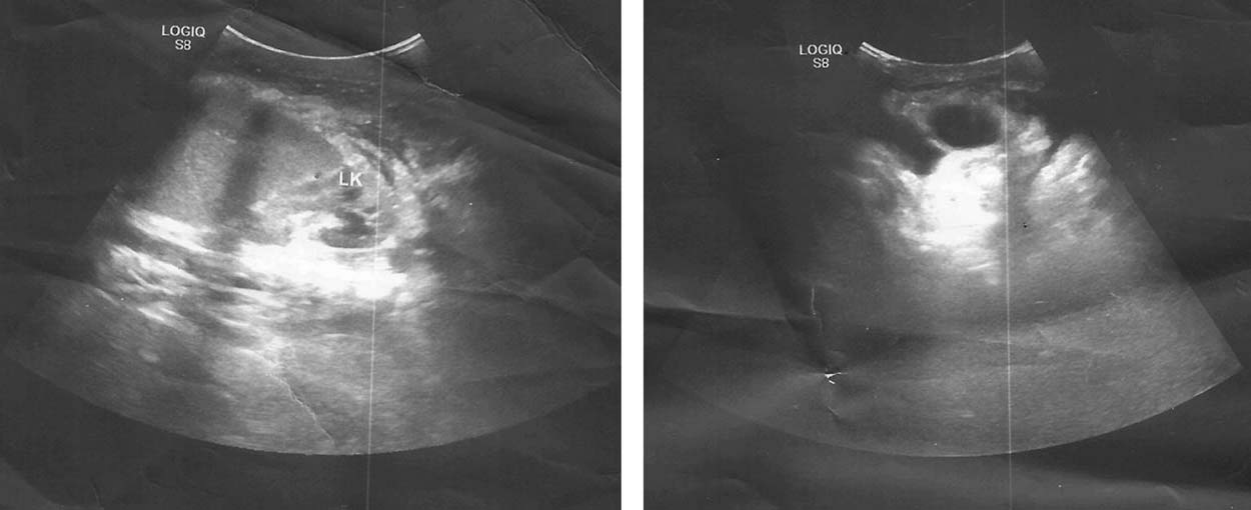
Ultrasound shows ascites and homogeneous splenomegaly.
